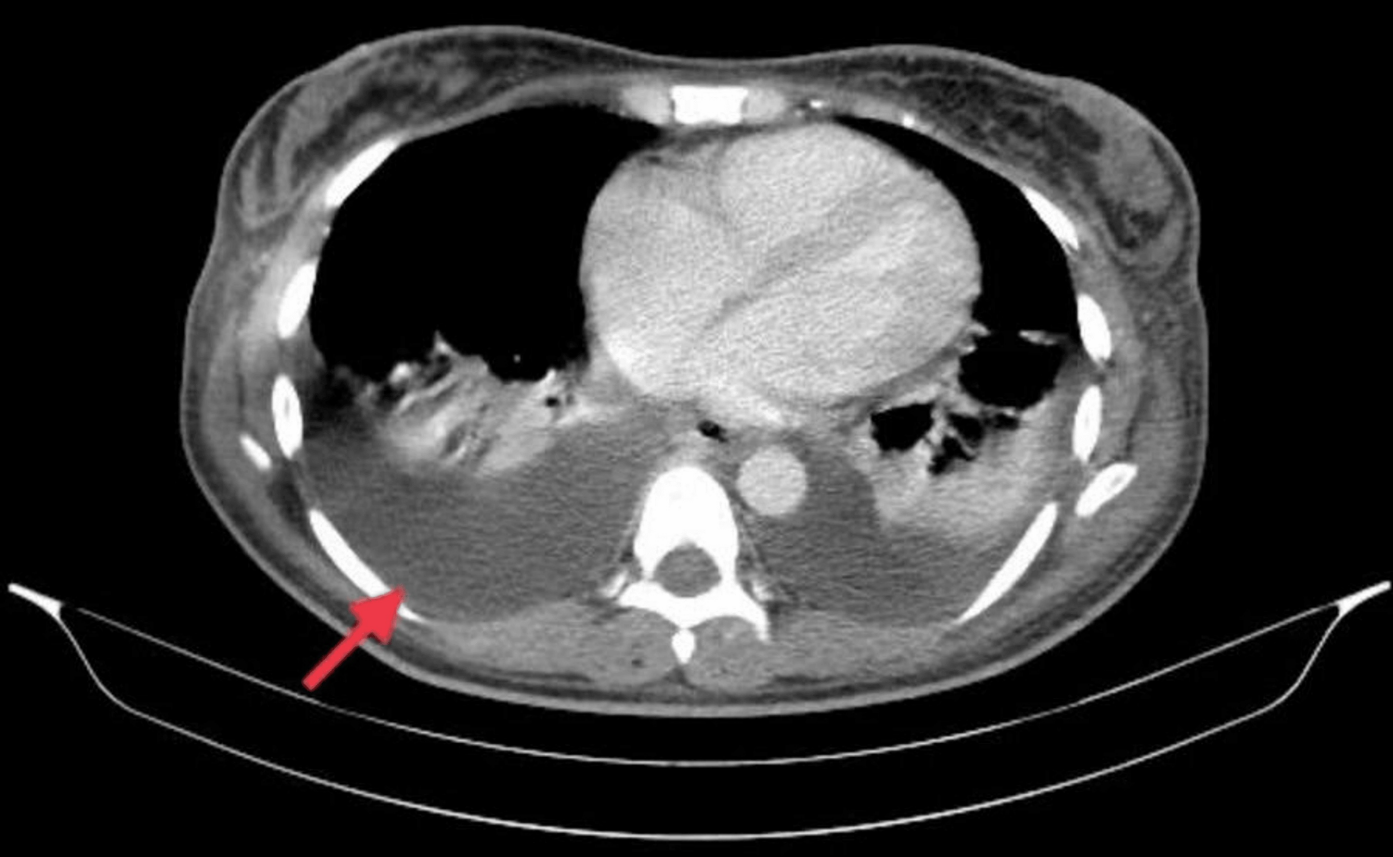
Chest CT scan revealed bilateral pleural effusions
